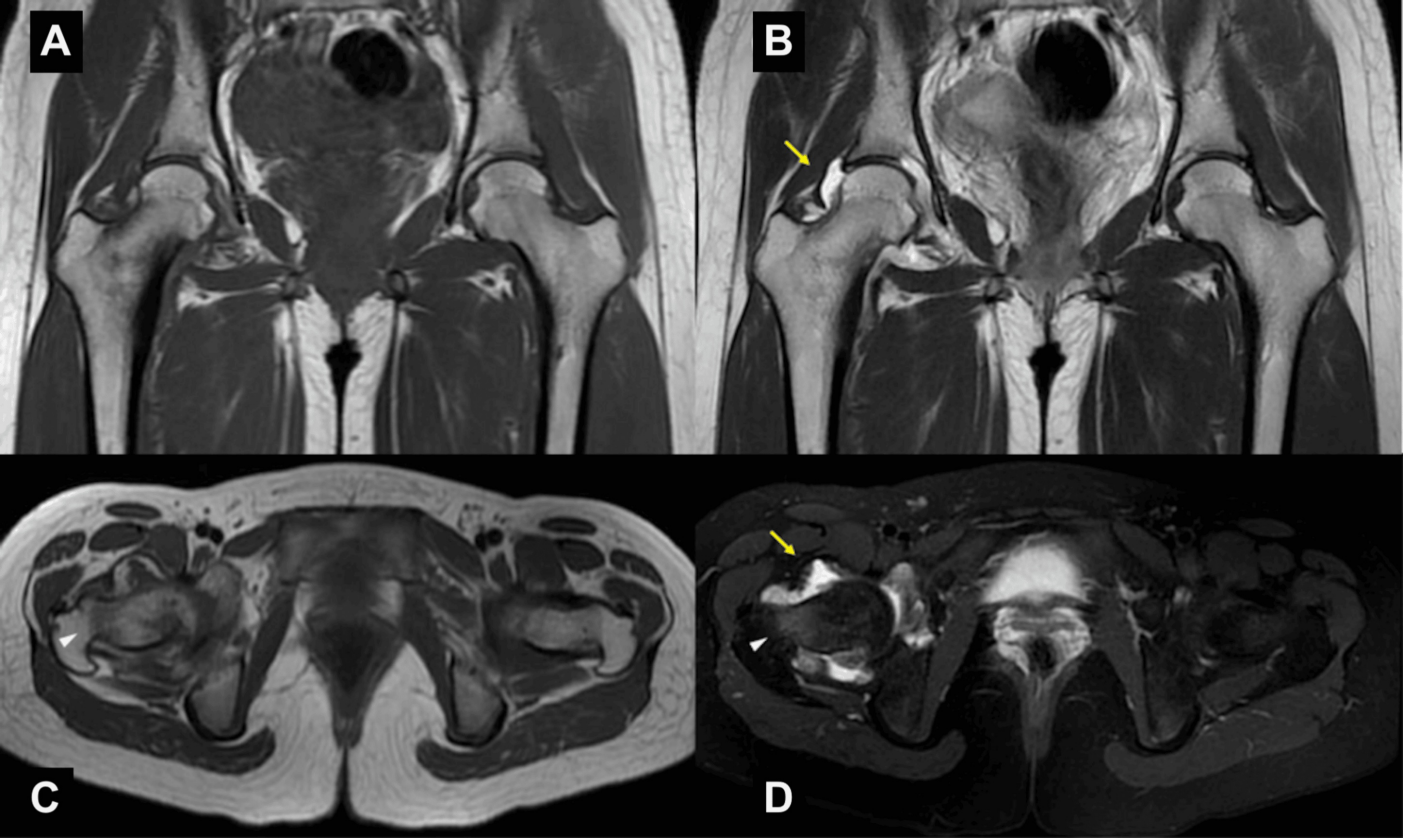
Preoperative magnetic resonance imaging (MRI) T1-weighted coronal/axial (A, C), T2-weighted coronal (B), T2-weighted fat-suppression axial (D). The yellow arrows show the joint effusion. The white arrowheads show the bone marrow edema at the femoral neck.
